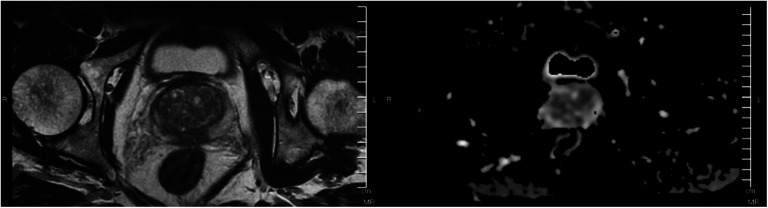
The MRI images of patient 1 (in Table 2). The T2W (T2-weighted) images and ADC (Apparent diffusion coefficient) images show bilateral paramedian lesions. All target biopsies were negative. The SB from the left showed 1/5 Gleason 4 + 4. This patient had a radical prostatectomy which showed multifocal Gleason 3 + 4. SB, systematic biopsy; MRI, magnetic resonance imaging.
